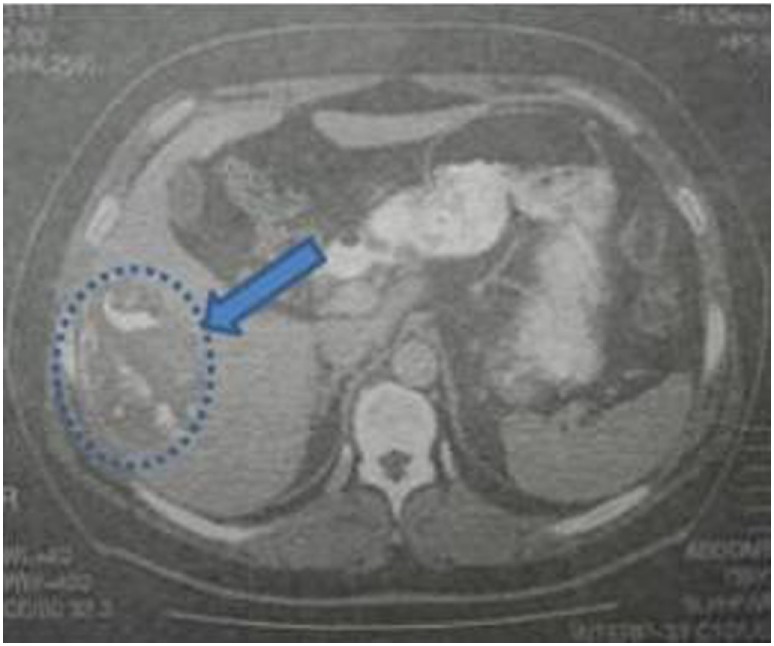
Abdominal computed tomography indicating a hepatic lesion with hyperdense walls, diffuse calcification, and foci of fat in the interior.
